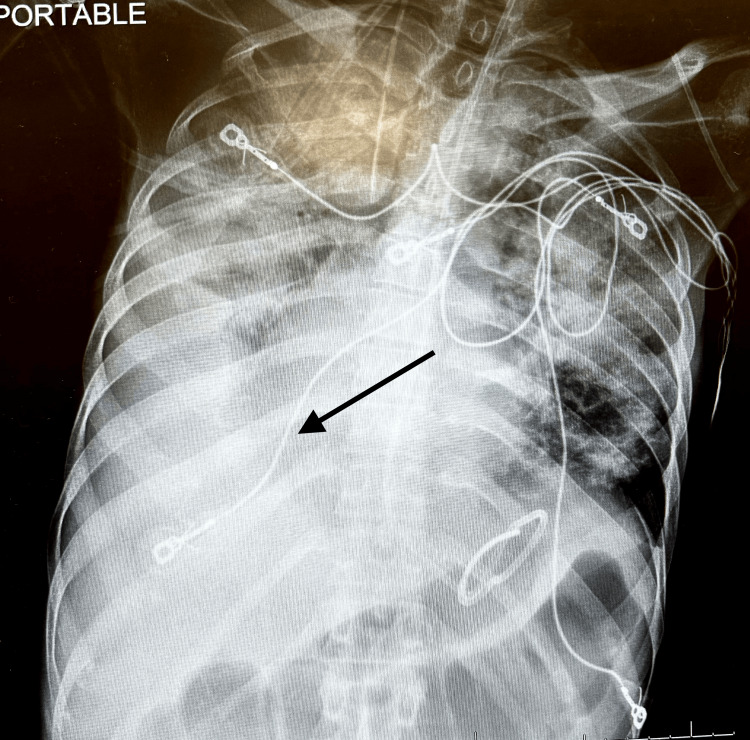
Chest X-ray The image displays a chest X-ray, and the arrow indicates a right-sided pleural effusion.
